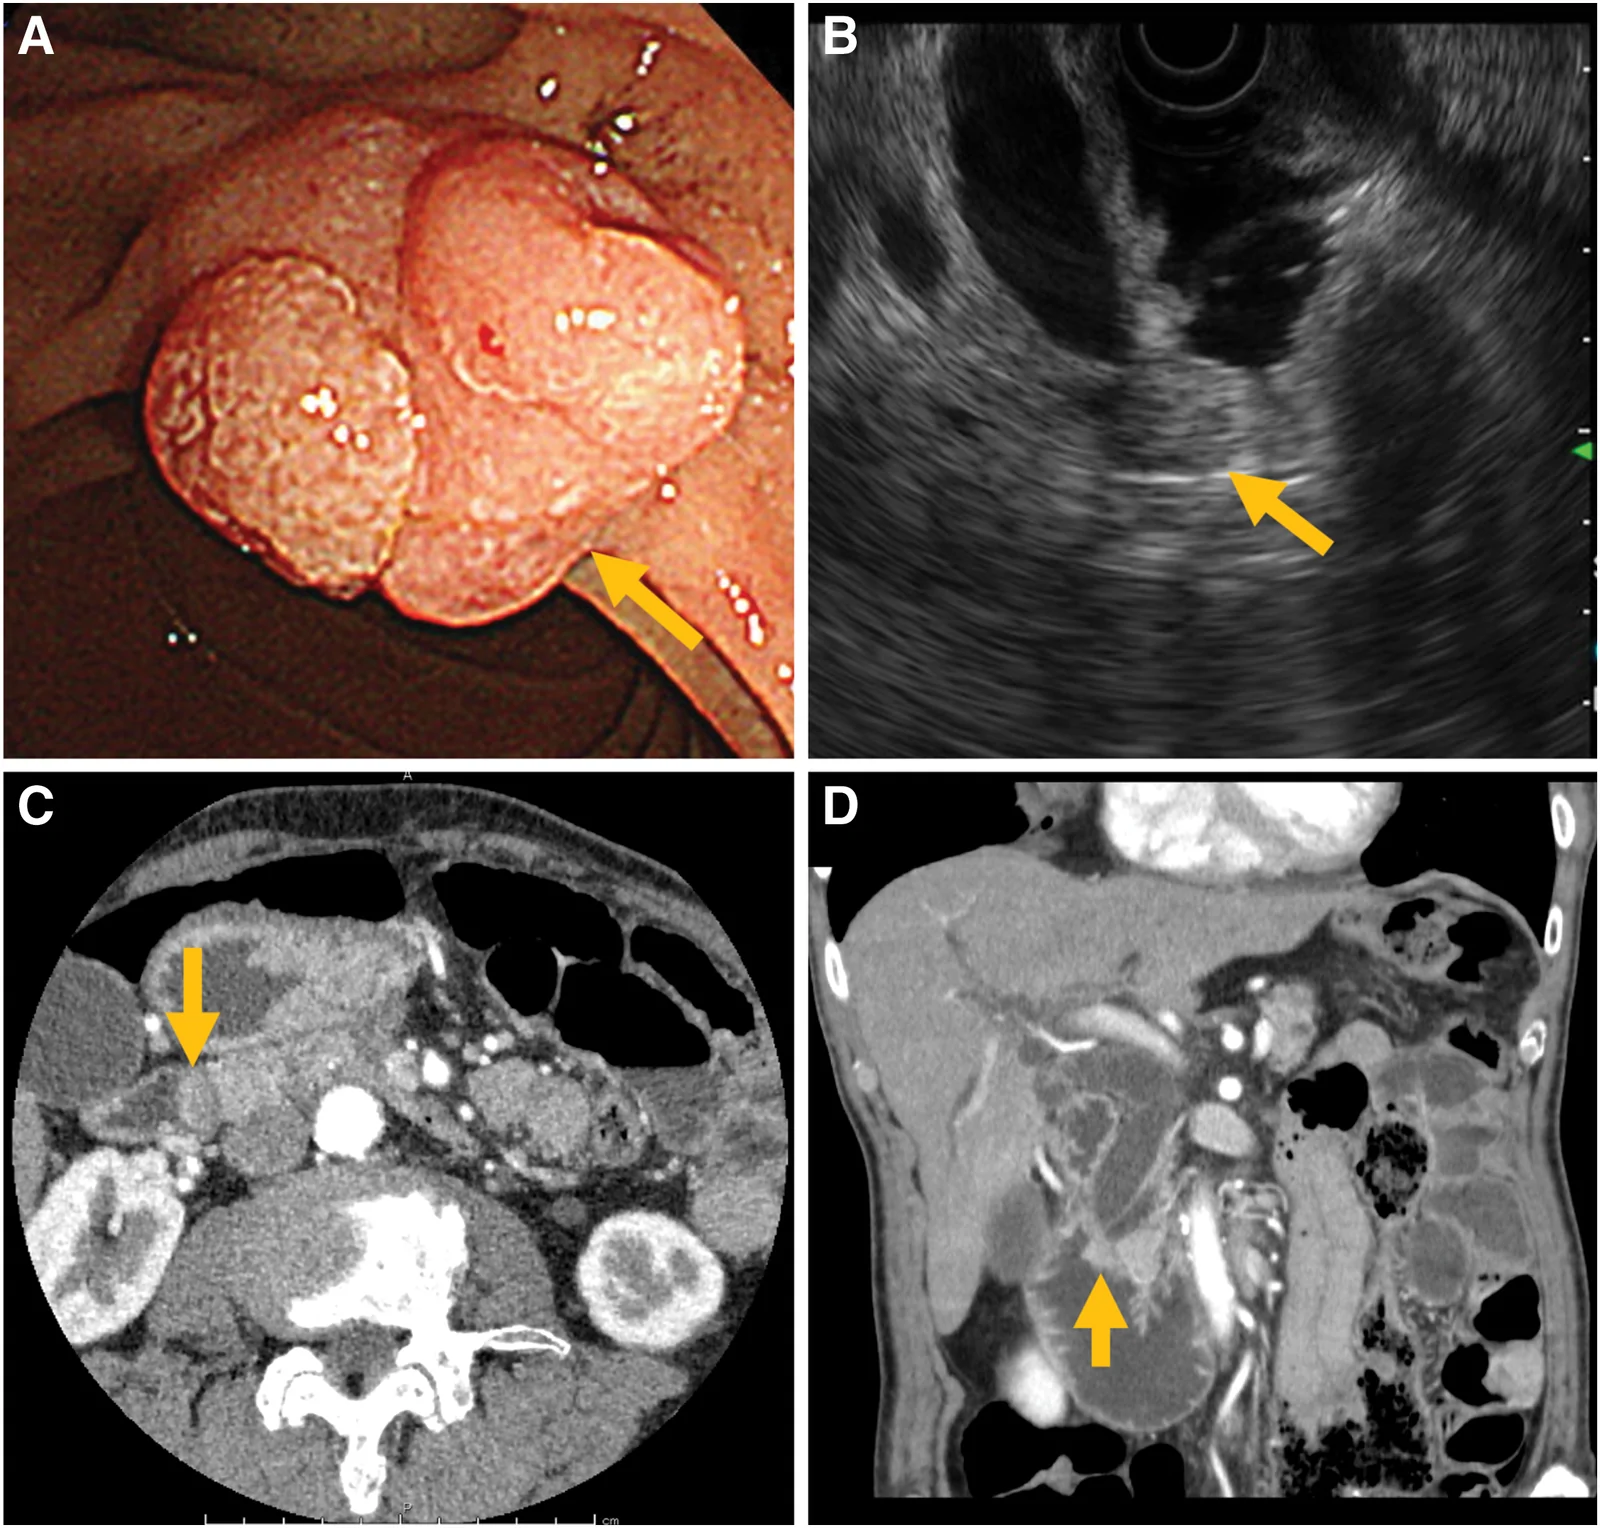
Preoperative endoscopic, endosonographic, and CT findings. (A) Upper gastrointestinal endoscopy showing an irregular elevated lesion at the ampulla of Vater (arrow). (B) Endoscopic ultrasonography showing a localized ampullary mass (arrow). (C) Contrast-enhanced axial CT showing a localized ampullary tumor (arrow). (D) Contrast-enhanced coronal CT showing the ampullary tumor (arrow) with upstream dilatation of both the common bile duct and main pancreatic duct. Although the biopsy revealed atypical epithelium suggestive of adenocarcinoma, the tumor size, irregular morphology, and double-duct dilatation raised concern for invasive ampullary carcinoma with possible extension into the distal bile duct and/or pancreatic duct. Therefore, PPPD with regional lymphadenectomy rather than endoscopic papillectomy was selected. PPPD, pylorus-preserving-pancreatoduodenectomy
